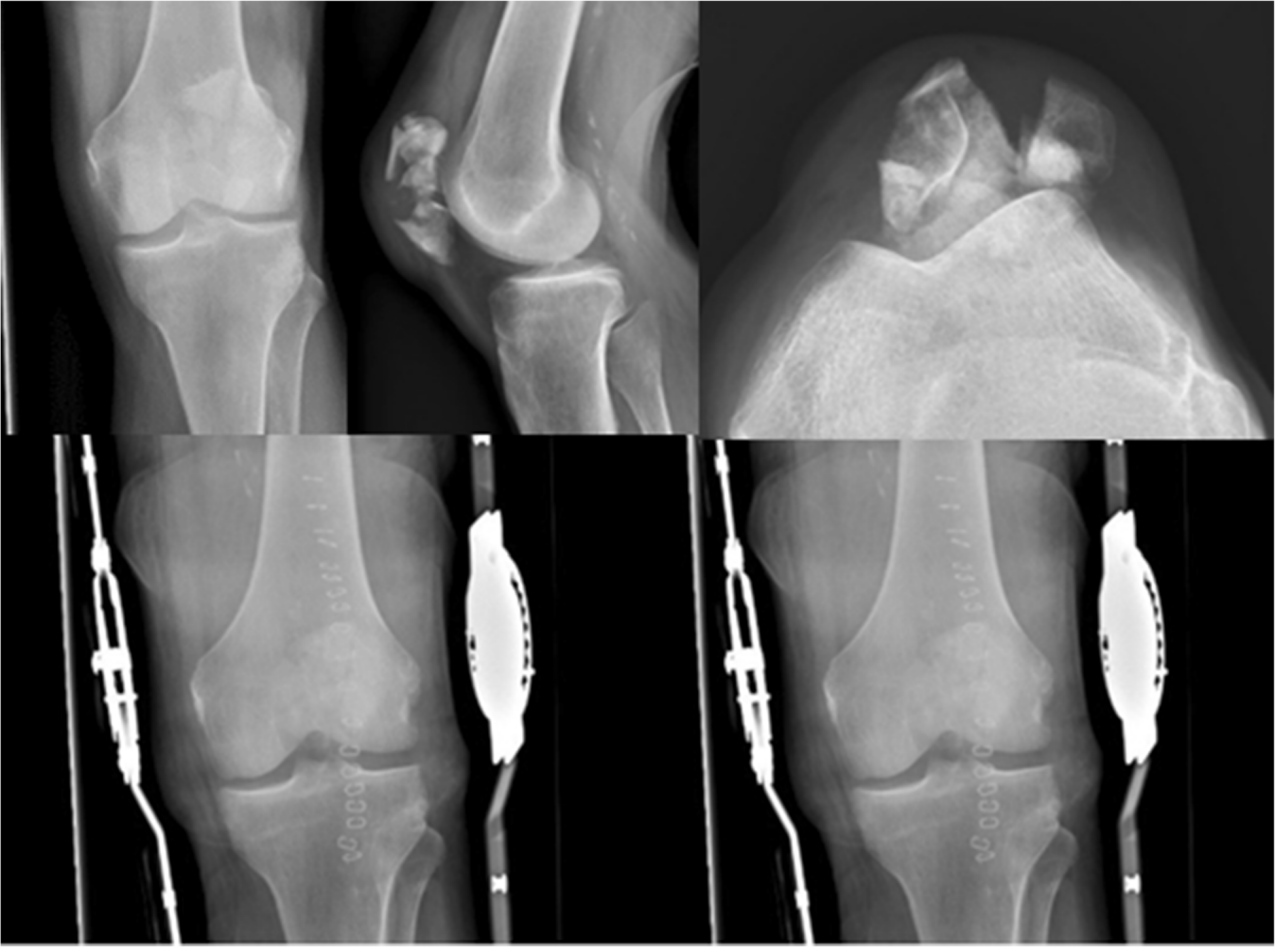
Pre- and postoperative X-rays (left and right, respectively) of patient 2, a 68-year-old man who presented with a severely comminuted left-side patella fracture
